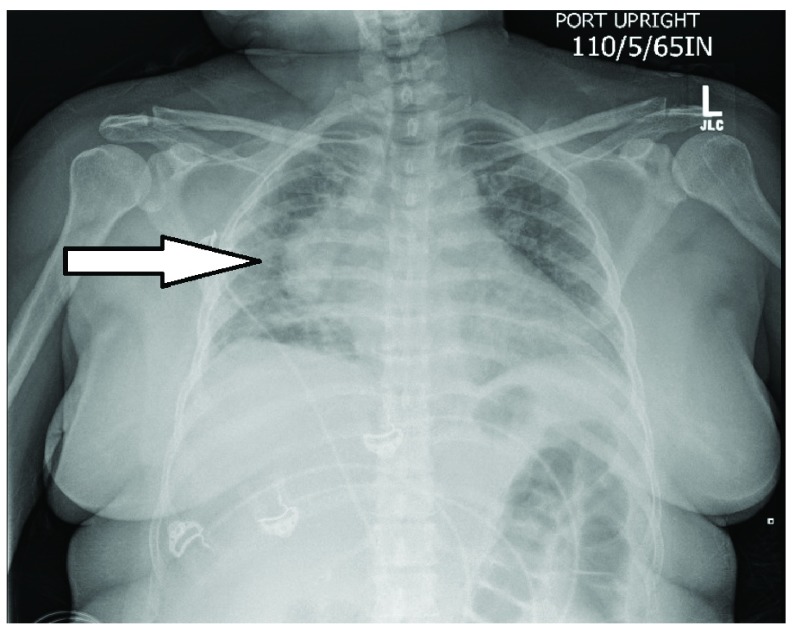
Chest X-ray showing right posterior mediastinal mass suggestive of a cyst (April 2017).
